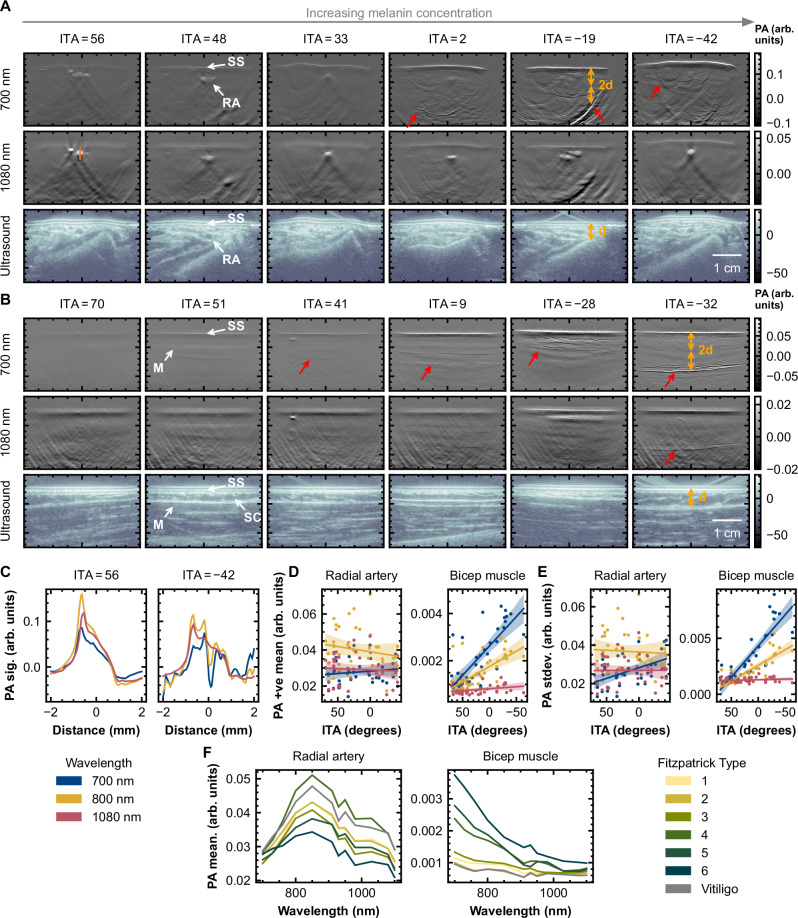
Single-wavelength photoacoustic data reveal increasing clutter signal with increasing skin pigmentation. Example ultrasound and photoacoustic images of the radial artery (A) and bicep muscle (B) at two wavelengths across a range of skin pigmentations, as measured with individual typology angle (ITA). White arrows label anatomical features: skin surface (SS), radial artery (RA), muscle (M), subcutaneous fat (SC). Red arrows show imaging artefacts; orange arrows demonstrate the depth, d, of anatomical structures and their corresponding artefacts. C Vertical lines plots of PA signal intensity through the radial artery in the subjects with the maximum ITA (least melanin, left) and minimum (most melanin, right) at several wavelengths. Mean, excluding negative pixels, (D) and standard deviation, including positive and negative pixels, (E) of the photoacoustic signal in the radial artery and bicep at several wavelengths as a function of ITA. Each point corresponds to one individual. The solid line shows a linear regression with a shaded 95 % confidence interval. Wavelengths shown in (C–E) are 700 nm (blue), 800 nm (orange) and 1080 nm (pink). F Mean photoacoustic spectrum (excluding negative pixels) for each Fitzpatrick type group and the vitiligo cohort in the radial artery and bicep muscle regions.
